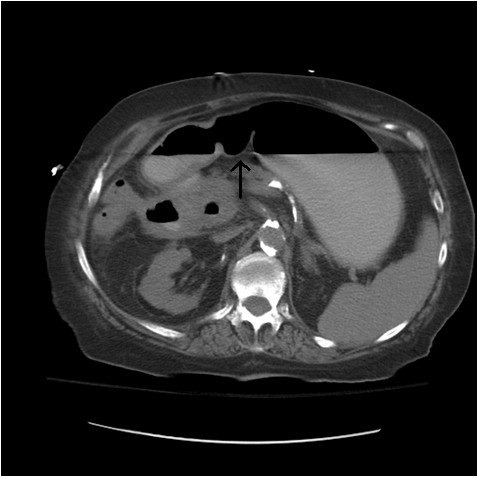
CT scan of the patient's abdomen showing gastric and duodenal dilatation (gallstone ileus).
